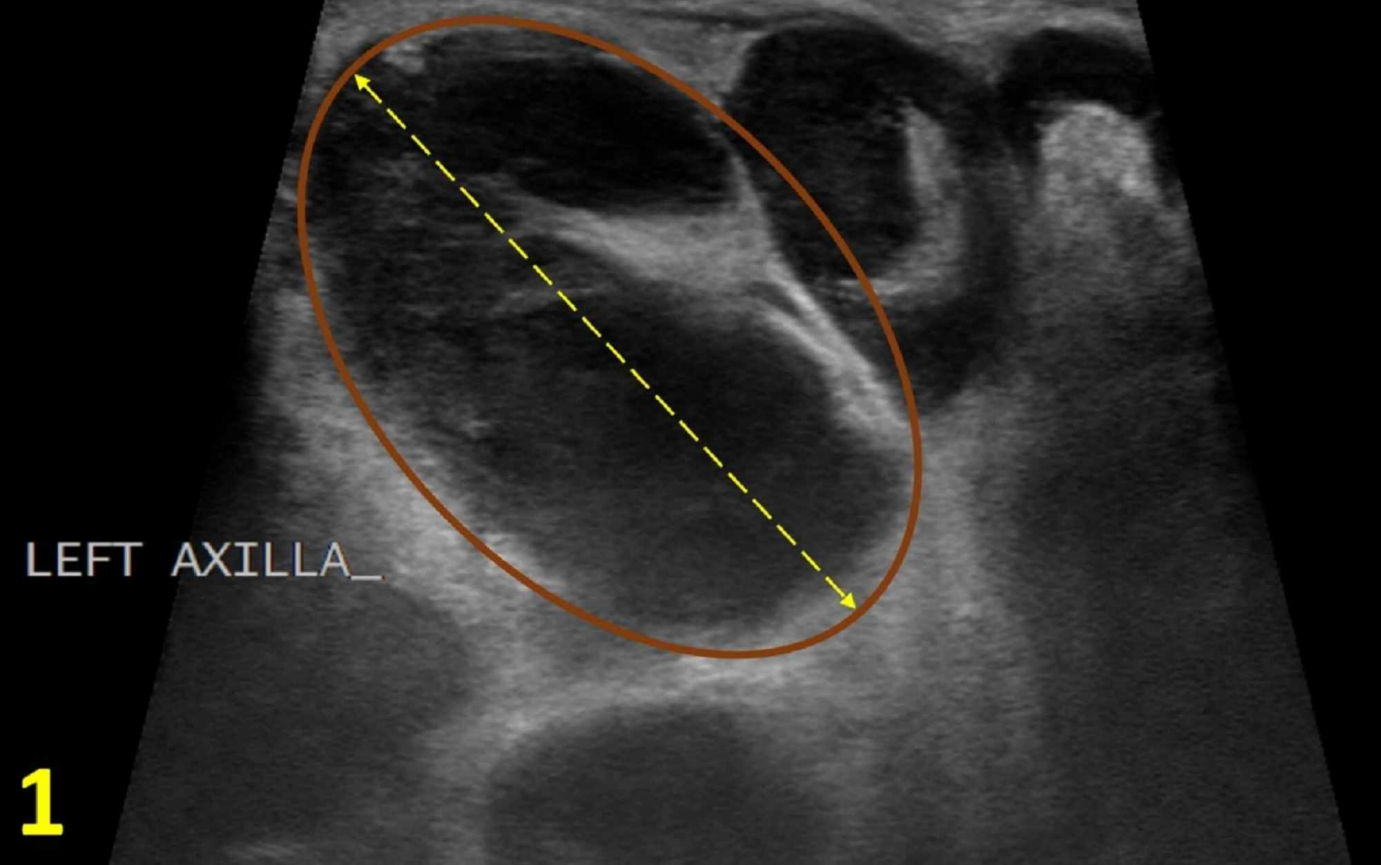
Left Axilla Diagnostic Ultrasound Enlarged axillary lymph node with cortical thickening (brown oval) measuring up to 33 mm (yellow double arrow).
